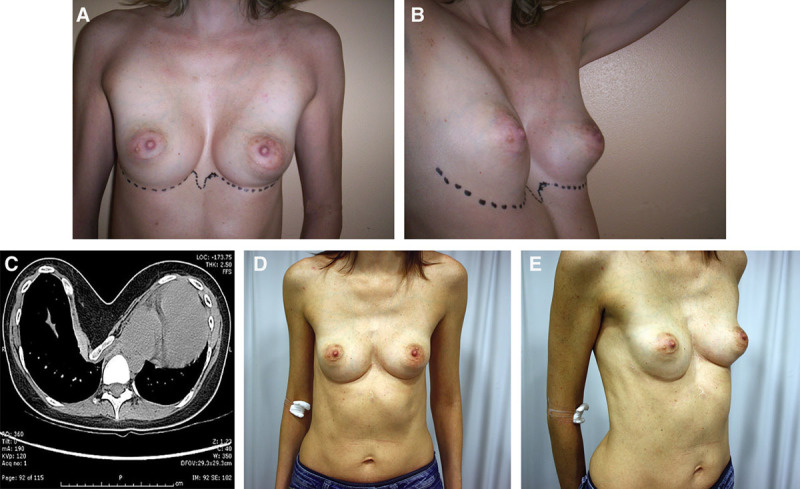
Case 1: A 30-year-old woman showing severe pectus deformity with functional impairment. A and B, Preoperative view of the patient. C, Preoperative CT scan of the chest showing limited anteroposterior diameter. D and E, Postoperative view at 18 months showing adequate and stable correction of the deformity.
